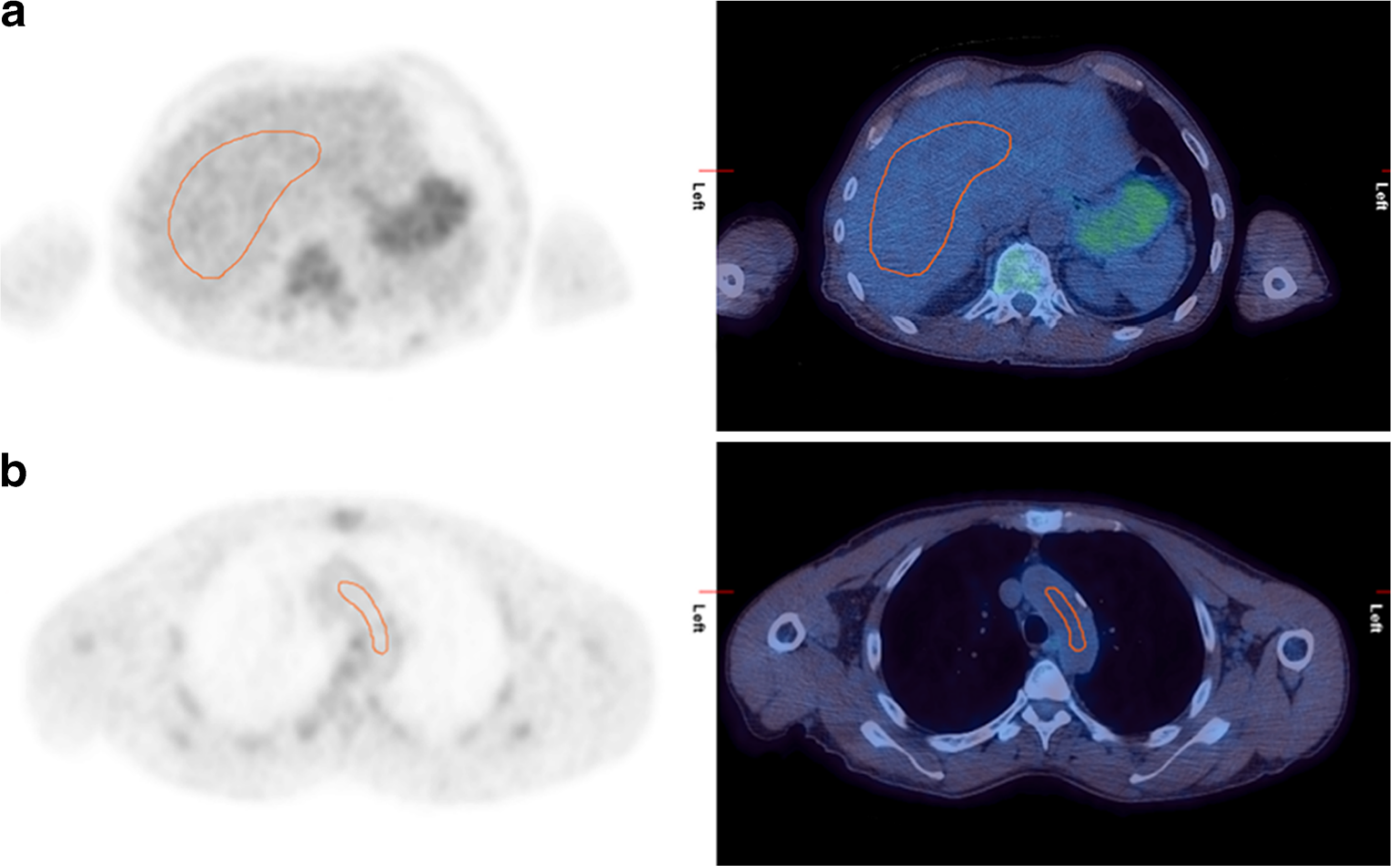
Axial slices demonstrating the St. Thomas’ method for assessment of maximum SUV within reference regions of the liver (a) and mediastinum (b) for confirmation of the Deauville score (courtesy of Dr. Victoria Warbey, PET Imaging Centre at St. Thomas’ Hospital London)
